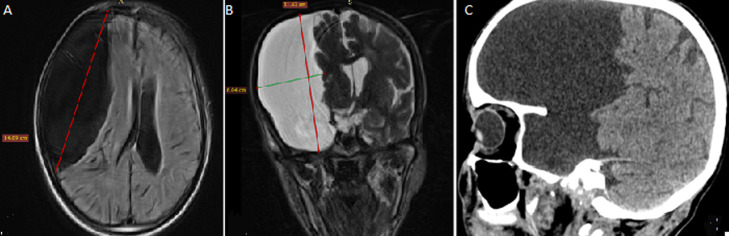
magnetic resonance imaging of the brain (axial T1-weighted; A) and coronal T2-weighted; B) cerebral computerizing tomography scan in spontaneous contrast, sagittal section; C) huge compressive arachnoidal cyst of the non-dominant hemisphere
